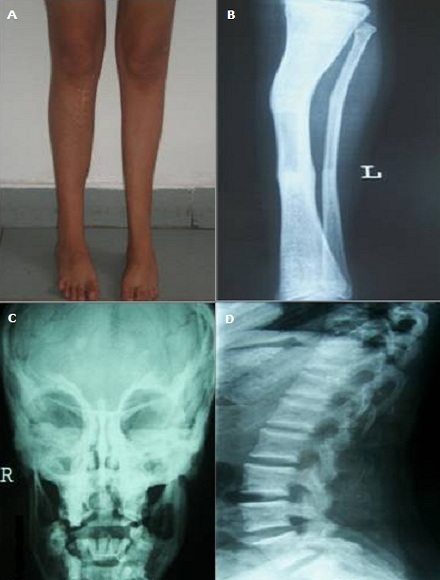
A) Déformations des os des membres inférieurs à type de genu valgum et pieds en varus équin à gauche et valgus du pied droit; B) Radiographies des membres inférieurs montrant des cals osseux avec du matériel d'ostéosynthèse; C) Radiographie de la face montrant un aspect en “loup de carnaval” du massif facial; D) Aspect de “vertèbres en sandwich” sur la radiographie du rachis lombaire de profil
